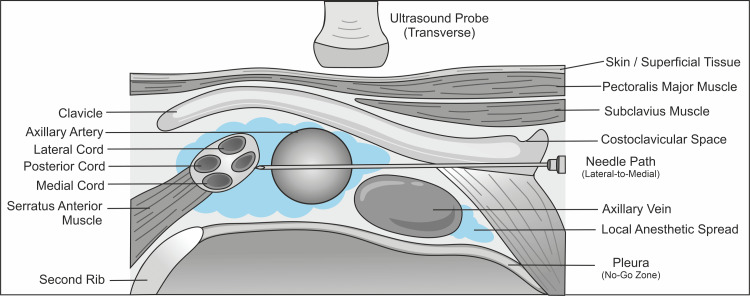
Anatomical and ultrasound-guided orientation of the costoclavicular brachial plexus block Created by the authors using CorelDRAW (Corel Corporation, Ontario, Canada)
